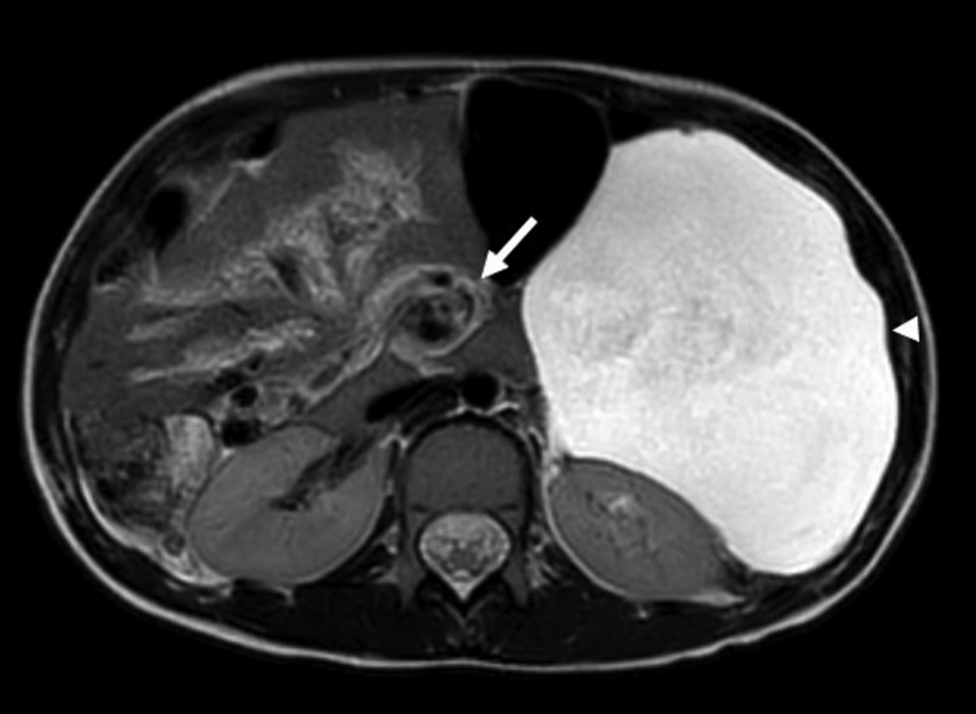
T2 magnetic resonance imaging sequence shows intra-abdominal fluid (arrowhead) and mesenteric rotation (arrow).
